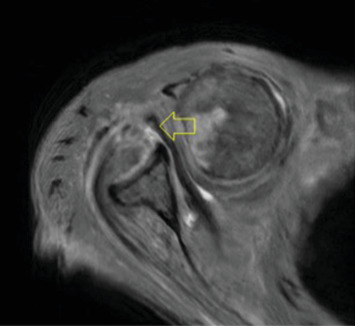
Axial T2-weighted MRI showing anterior dislocation of the right humeral head; the yellow arrow demonstrates interposition of the subscapularis tendon between the humeral head and glenoid.
